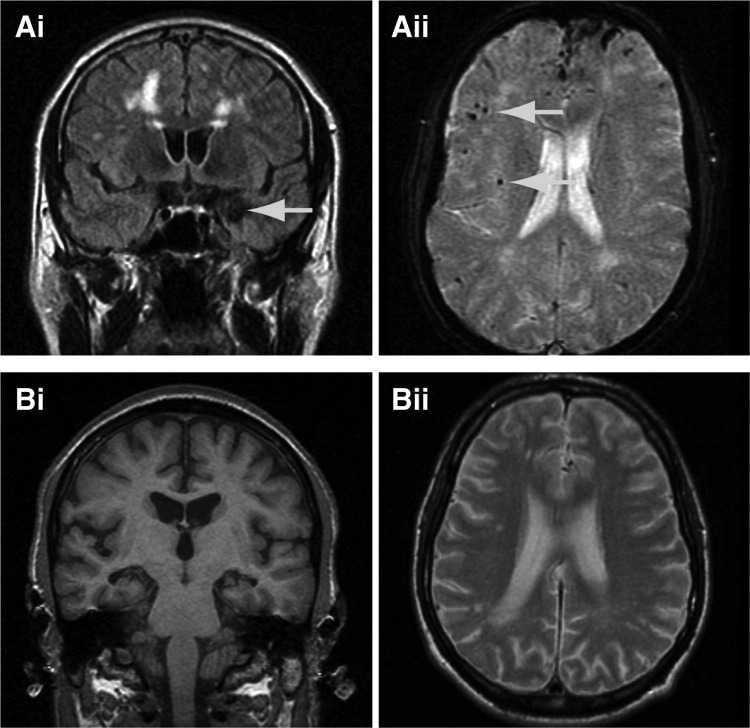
Magnetic resonance imaging (MRI) scans from *APP*dup patients. (A) Proband 1 showing extensive white matter abnormalities, microbleeds, (Aii) and an amygdala hemorrhage (Ai, arrow), and (B) proband 2 (aged 47) showing white matter abnormalities on T2 weighted scans (Bii) and hippocampal atrophy (Bi).
